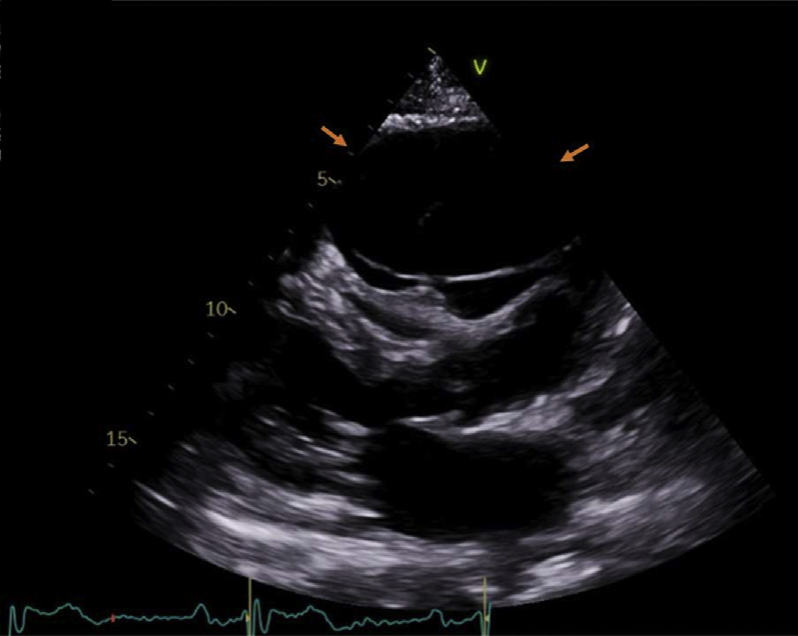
Transthoracic Echocardiogram Shows Anechoic Septated Collection Obstructing the Right Ventricular Outflow Tract
